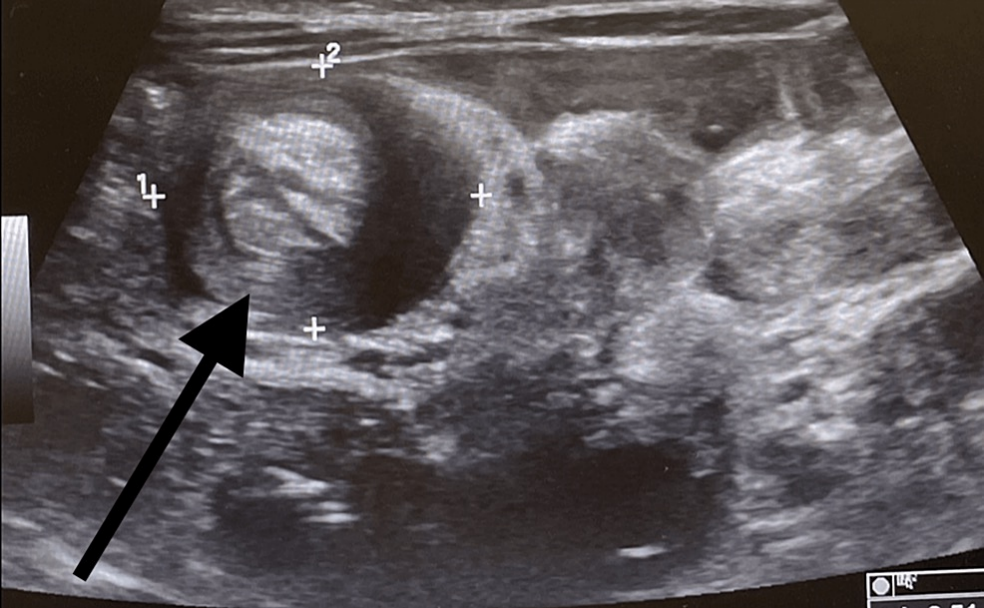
Day 3 abdominal ultrasound image showing the target sign in the right upper quadrant
